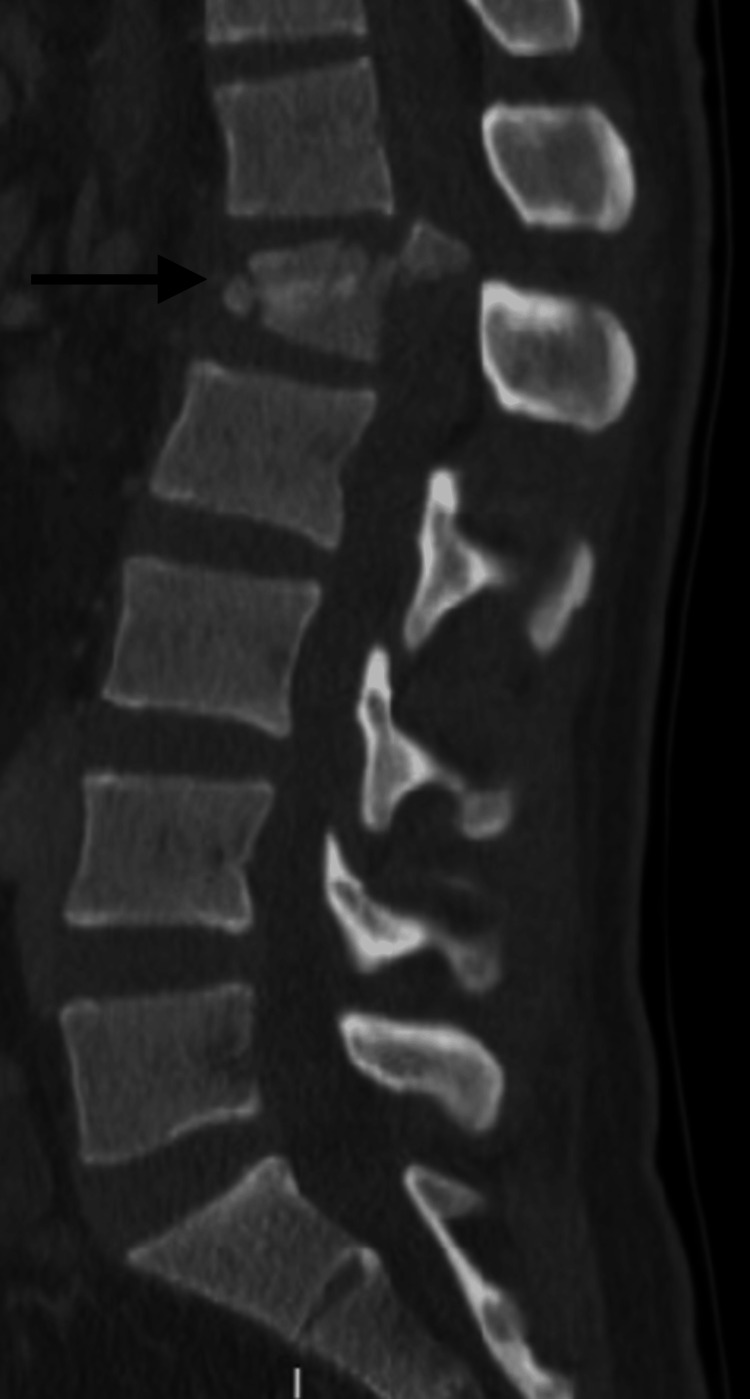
Preoperative sagittal CT of patient 2 shows a complete burst fracture (AOSpine subtype A4) of L1 (black arrow) with significant retropulsion.
